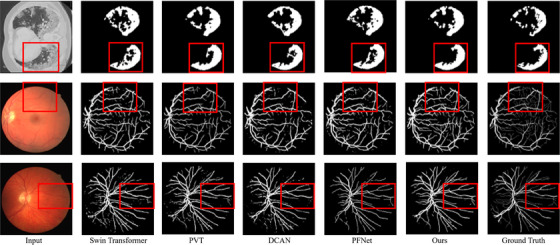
Partial visual results of our method, as well as several other fully supervised and semi‐supervised learning models, on the COVID‐19 CT test set and DRIVE test set.
